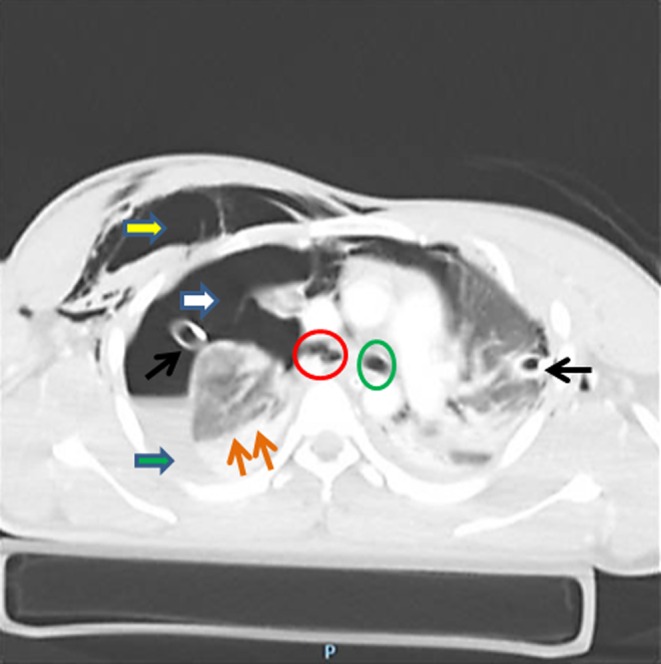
Axial plane view of the CT chest on lung window shows right‐sided subcutaneous emphysema (yellow arrow), right pneumothorax (white arrow), right hemothorax (green arrow), “fallen lung” sign (double brown arrows), and the presence of bilateral chest tubes (black arrows). The left main bronchus silhouette is intact (green circle), while the outline of the right main bronchus is disrupted (red circle). The above radiological findings are highly suggestive of right main bronchus rupture. A bedside bronchoscopy confirmed the diagnosis
